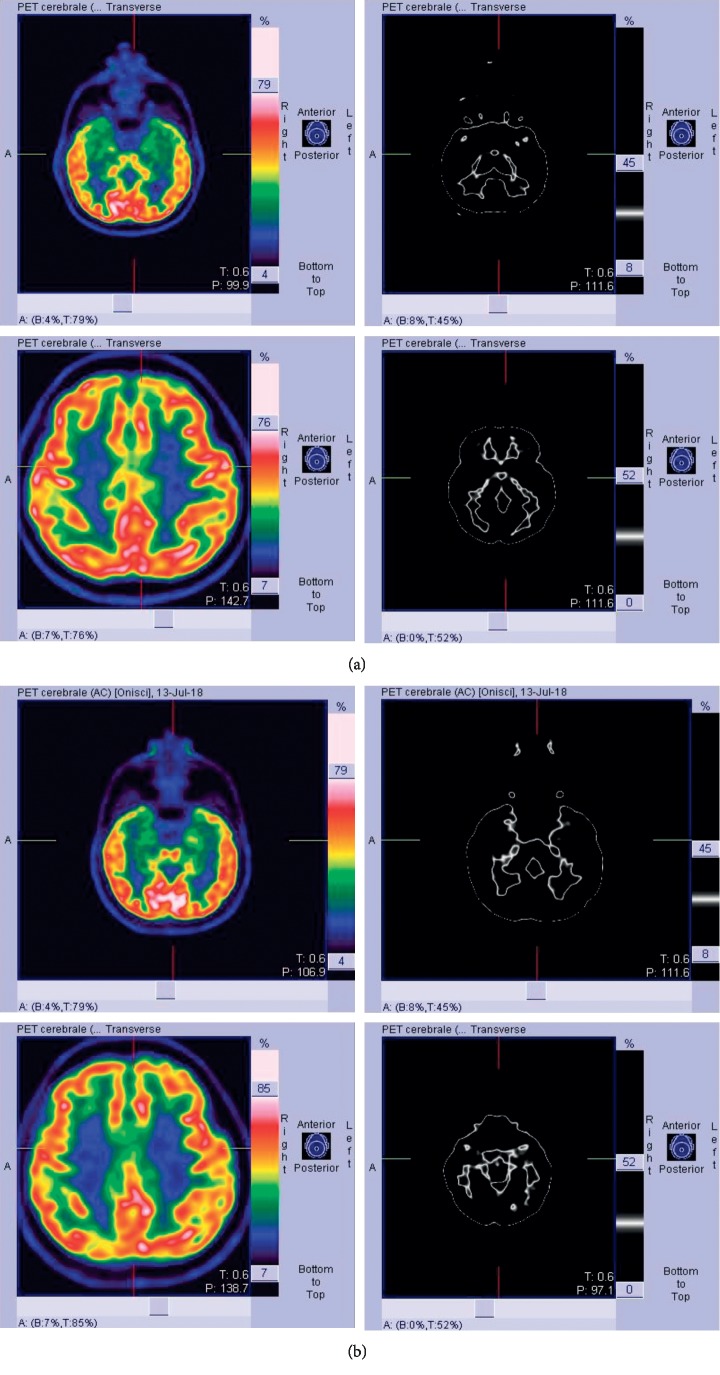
[^18^F] fluorodeoxyglucose positron emission tomography ([^18^F]-FDG PET) showed glucose metabolic alterations in both temporal lobes, mainly involving the mesial regions, and in the left insular and parietal regions.
